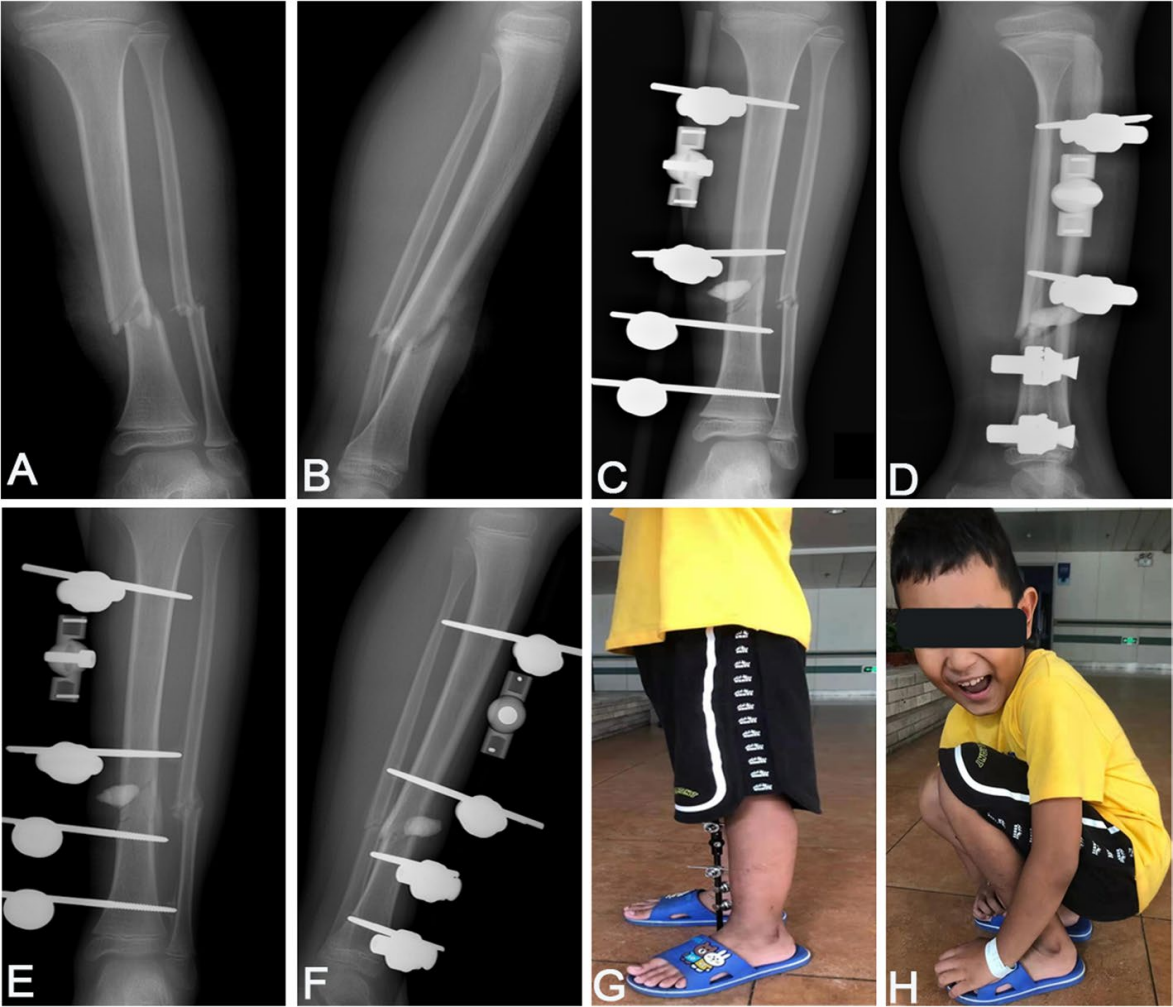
Representative cases with Gustilo type I open tibia-fibula shaft fractures at preoperation and postoperation. A, B X-ray images of a 7-year-old boy at preoperation. C, D X-ray images at postoperative immediate showed good reduction and use of local antibiotic bone cement to prevent infection. E, F X-ray images at postoperative 1 month. G, H At 4 weeks postoperatively, the patient walked without crutches and recovered well
